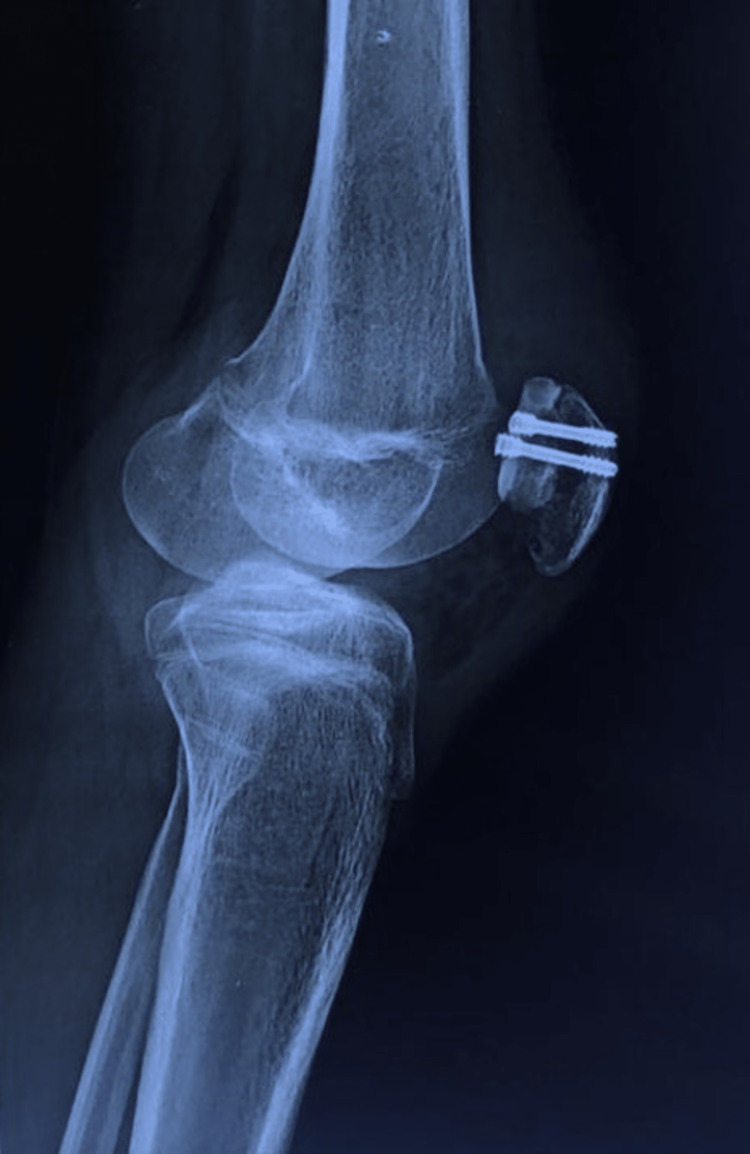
Postoperative lateral roentgenogram
